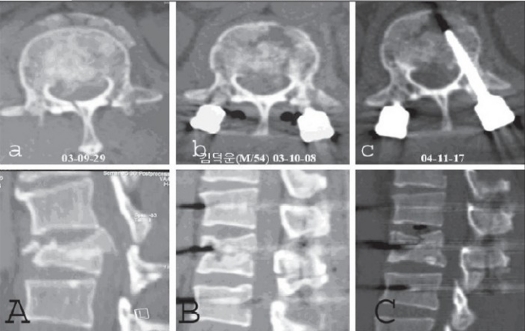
L_1_ burst fracture with retropulsed fragment: (aA) Preoperative CT shows vertically collapsed upper 1/2 of L_1_ body with a retropulsed fragment and (bB) well-reduced fracture is seen in postop CT. (cC) Fracture consolidated on 13 months followup
